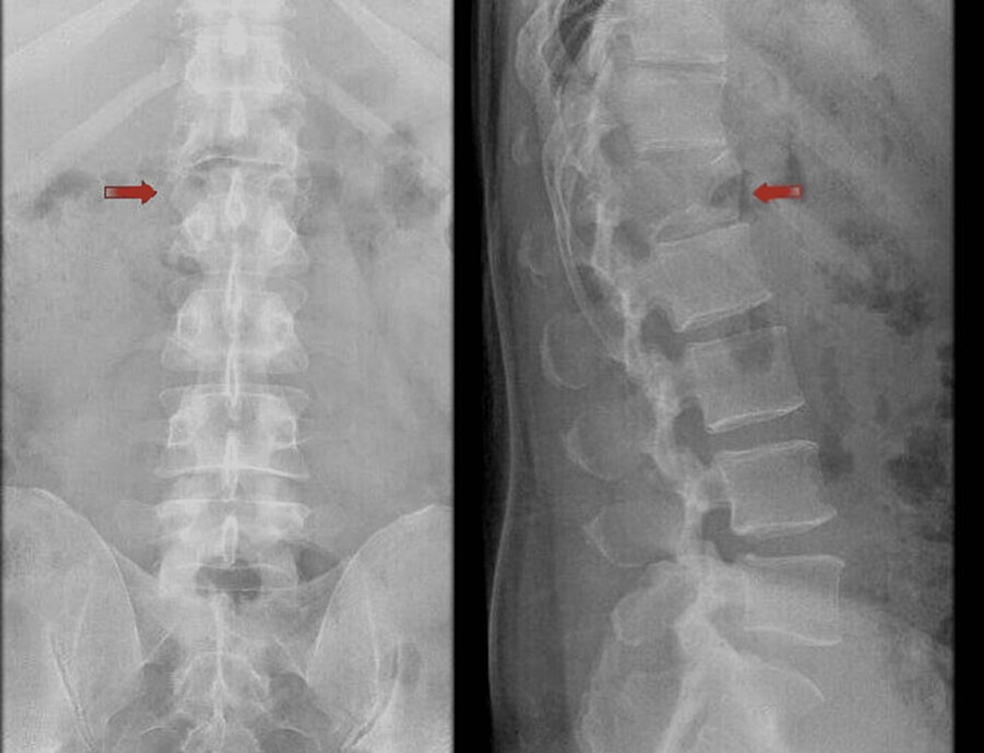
Lateral and anterior-posterior lumbar X-ray demonstrating L1 fracture
